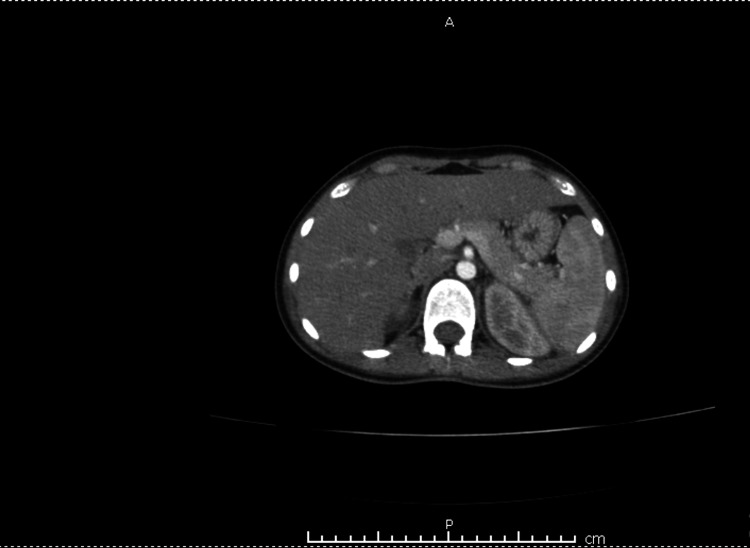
Abdominal CT showing hepatosplenomegaly with embolic showers
